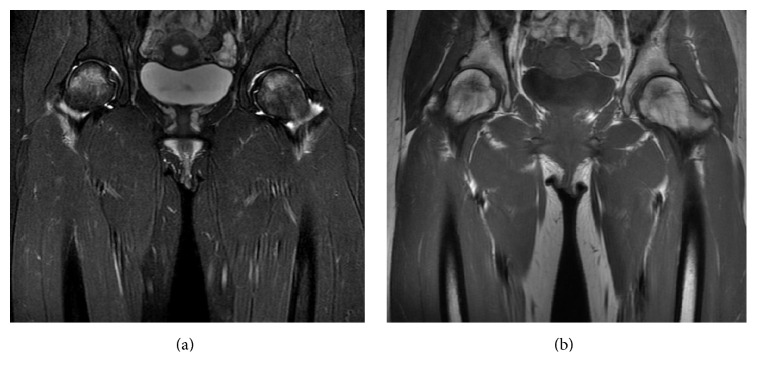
Magnetic resonance images of pelvis one week after symptom onset. (a) Coronal T2-weighted magnetic resonance image. Increased signal uptake within the femoral heads and necks bilaterally. There were no signs of osteonecrosis. (b) Coronal T1-weighted magnetic resonance image. Decreased signal uptake within the femoral heads and necks bilaterally.
